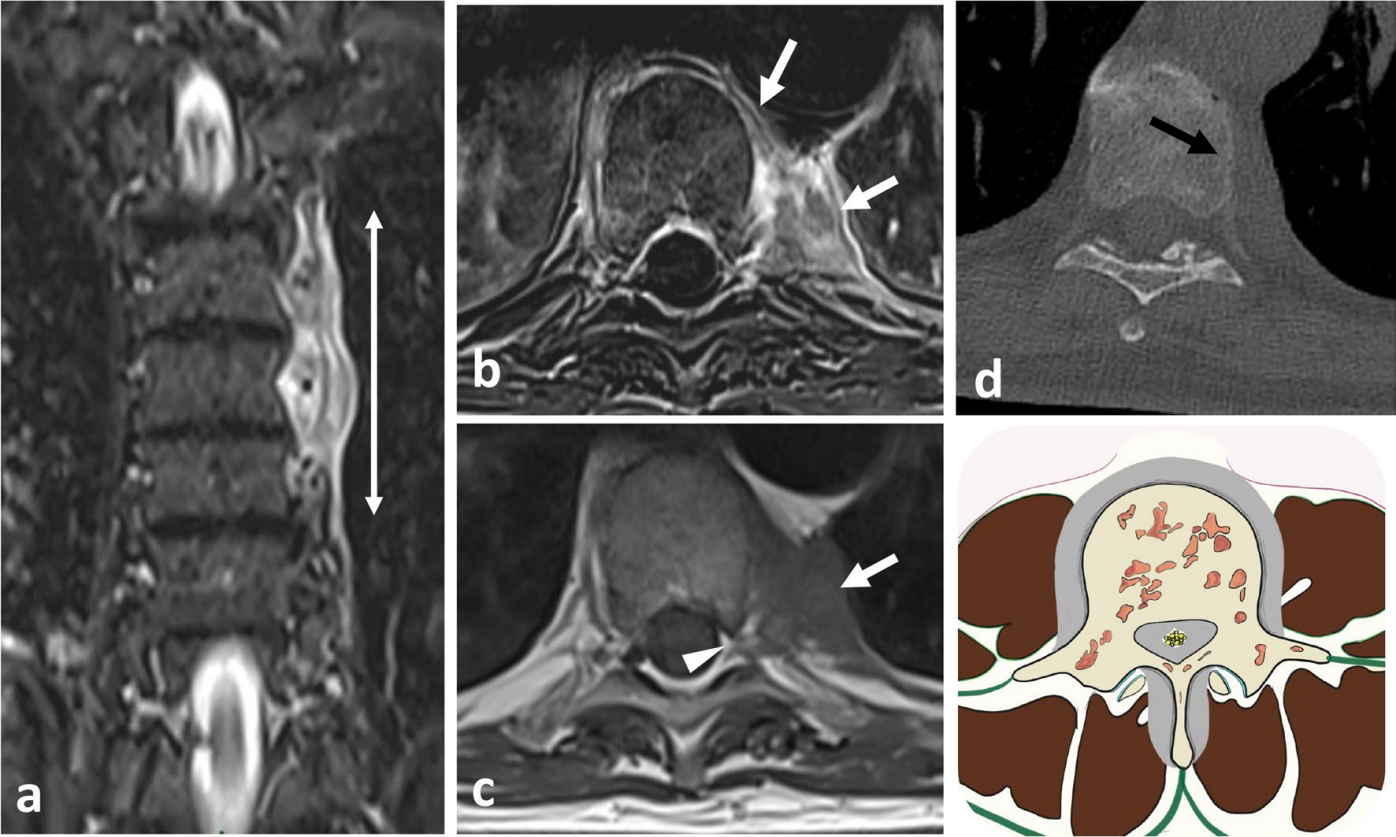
Non-Hodgkin lymphoma* in a 72-year-old male with fatigue and mild thoracic pain at rest. Coronal STIR (a) shows left paraspinal infiltrative mass along three vertebral segments (double head arrow). Axial T1WI (b) and subtraction T1WI (c) shows an epaxial mass (white arrows), which extents on the ventral surface of the vertebral body and penetrates into the vertebral foramen (arrowhead). Both MRI and CT (d) demonstrate that the mass do not invades the vertebra (black arrow)
